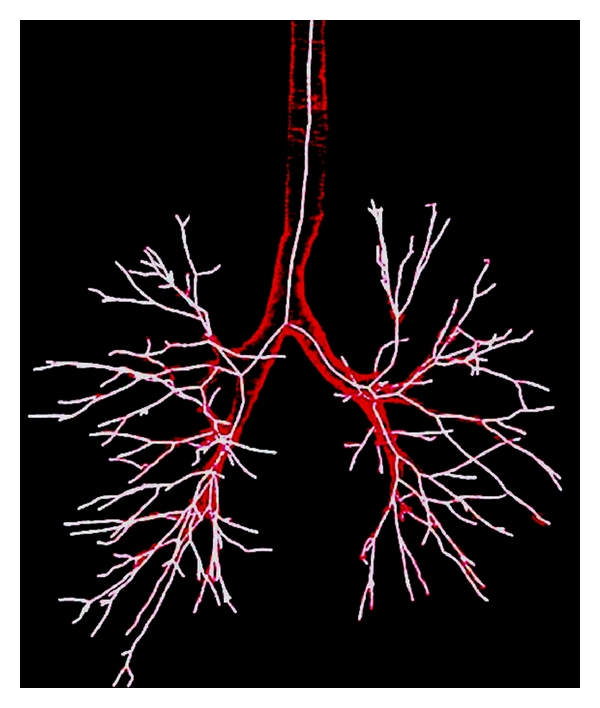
Bronchial tree volume automatically segmented using homemade dedicated software, extracted from a whole set of lung CT images. The skeleton of the bronchial tree is computed to obtain a simplified three-dimensional geometry of the bronchial tree.
